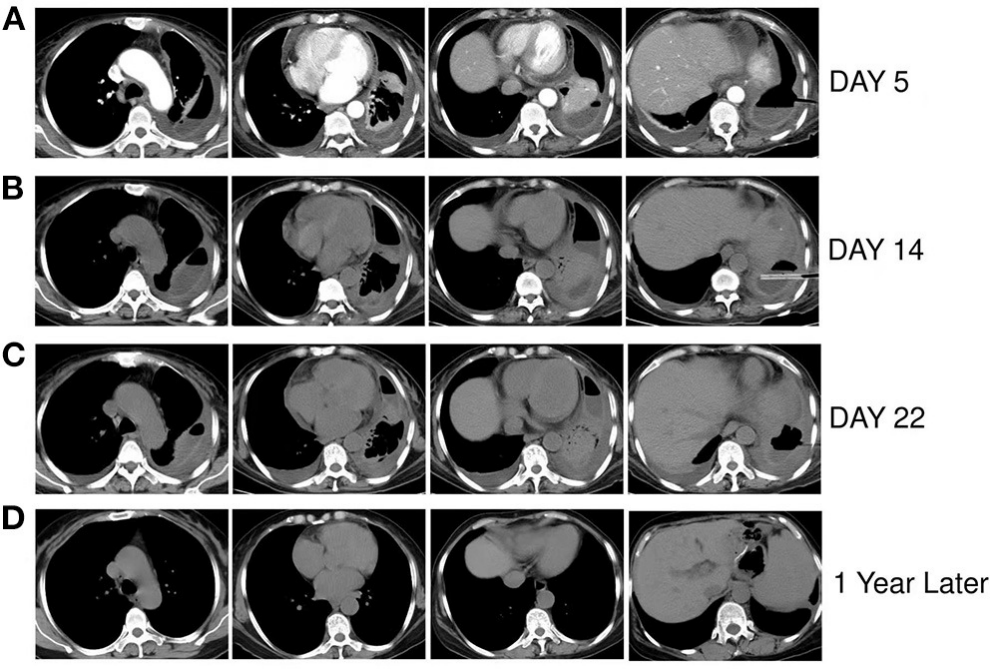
(A–C) Are respectively the chest CT scans of the patient on the 5th, 14th, and 22nd days of hospitalization, showing the left lung was gradually re-expanded, the left pleural effusion was gradually reduced, and the left pleural thickening was gradually decreased. (D) Chest CT of the patient nearly 1 year after discharge, showing that the left lung recruited, a very small amount of pleural effusion on the left side and slightly thick pleura.
